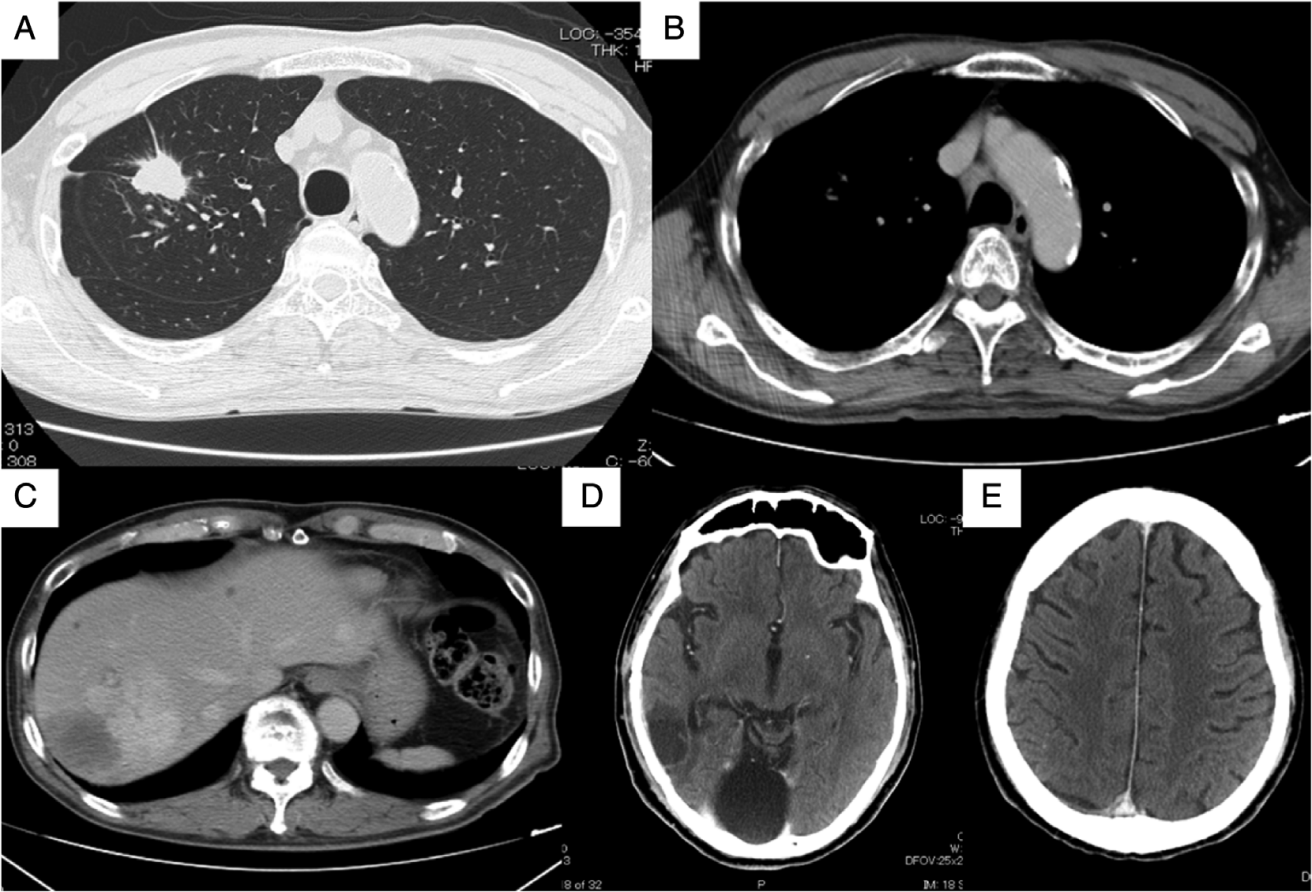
Systemic contrast‐enhanced computed tomography (CT) images at 1 month after starting alectinib. (A,B) Chest CT showed shrinkage of the lung carcinoma to 24 mm and of the metastatic lesions in the mediastinal lymph nodes. (C) Abdominal CT showed shrinkage of the liver metastases. (D,E) Head CT showed that the brain metastases in the right parietal lobe had disappeared completely. Brain metastases in the right occipital lobe were after surgical resection, so cannot be included in the evaluation of the therapeutic effect of alectinib
